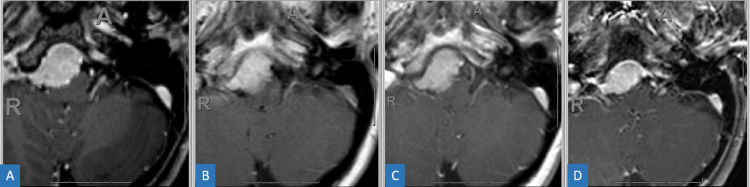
Pre-treatment (A), four-month (B), one-year (C), and two-year (D) post-treatment axial T1 post-contrast MRI of a skull-based WHO grade I meningioma. Notice the hazy tumor edges on four-month and one-year follow-up scans with eventual contraction of the tumor at two years. MRI = magnetic resonance imaging; WHO = World Health Organization
